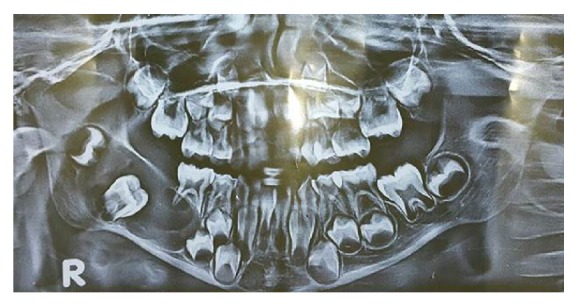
Orthopantomogram reveals radiopaque lesion surrounded by a radiolucent zone extending anteriorly from the lower first molar region to body of the mandible posteriorly on the right side.
